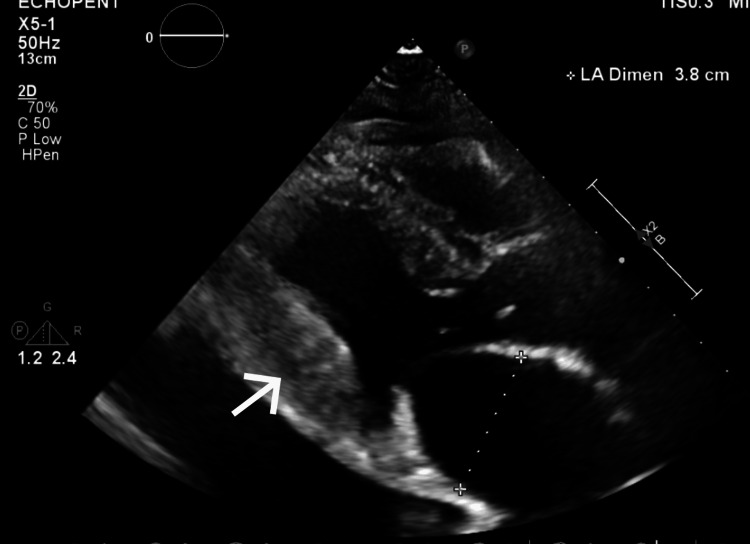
Transthoracic echocardiogram demonstrating a grainy appearance to the myocardium, as denoted by the white arrow This interpretation by the cardiologist first raised the clinical suspicion for amyloidosis. This finding, along with the increased left atrial enlargement (demarcated by the dotted line) and the corresponding left ventricular hypertrophy (also denoted by the arrow), showed a pattern typically seen in amyloidosis.
